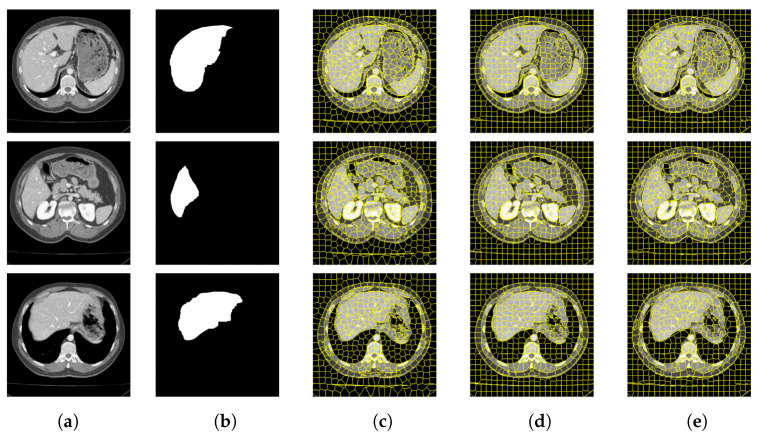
CT image segmentation of liver. (a) Original image. (b) True image. (c) SLIC. (d) SNIC. (e) Proposed.
